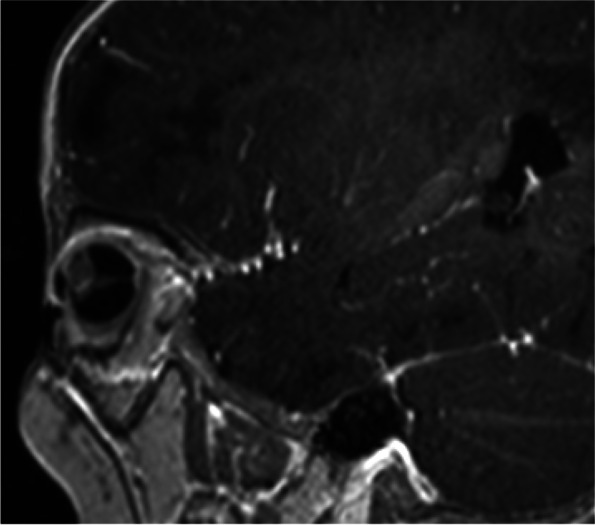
MRI orbits axial T2 and sagittal post-contrast T1 sequences demonstrate right microphthalmia, a T2 intermediate signal mass associated with the lens, extending along a linear structure to the optic disc (Cloquet’s canal). The right lens is small and T2 intermediate signal when compared to the left
